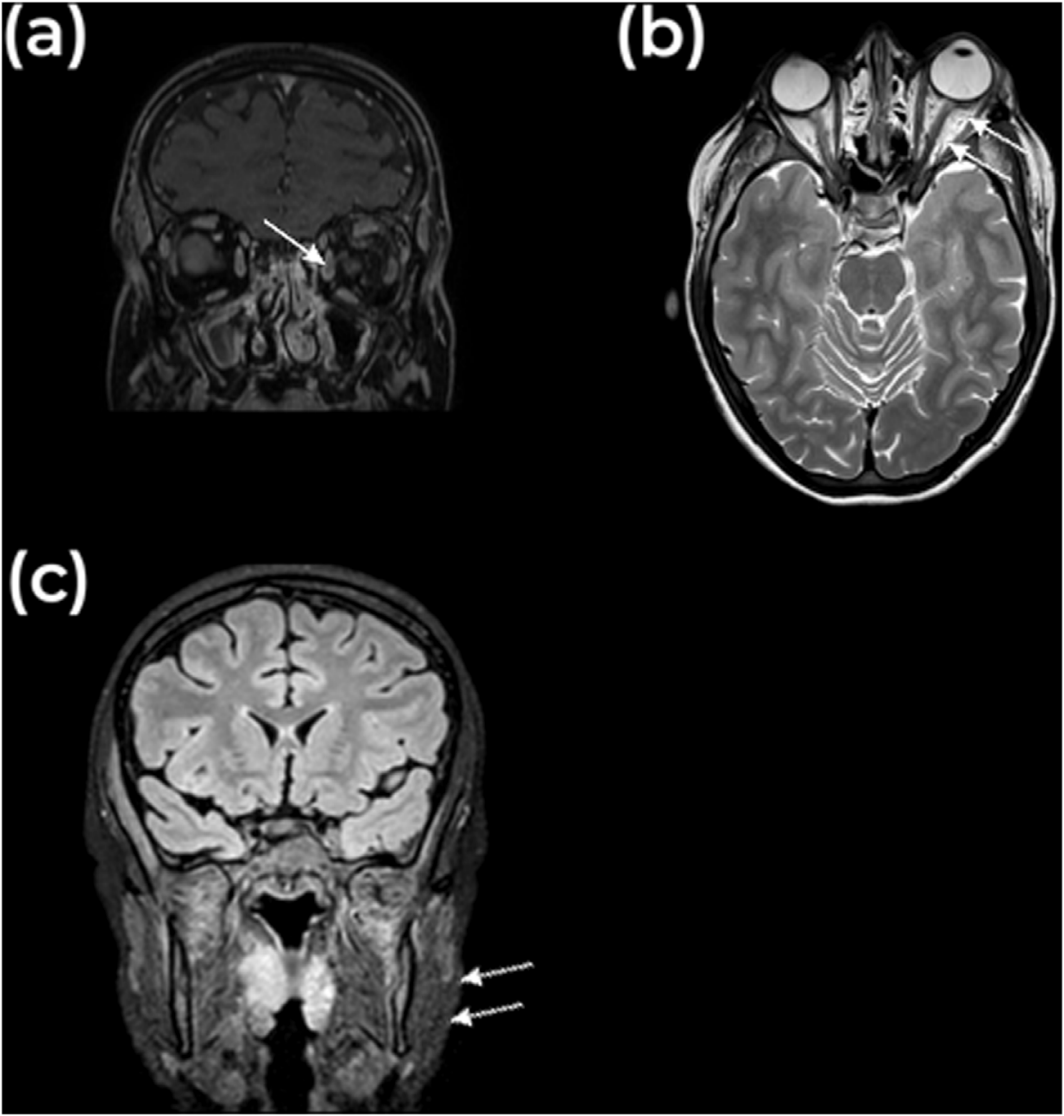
MRI findings. (A) Coronal and (B) Axial view of MRI image, T1 weighted, showing axial proptosis with infiltration of the intraconal fat and a non-perfused enlarged superior ophthalmic vein, indicated with the arrow. The superior lateral and medial rectus muscles are slightly enlarged. (C) Coronal view, showing enlargement of the masticator muscles, indicated with the arrow.
